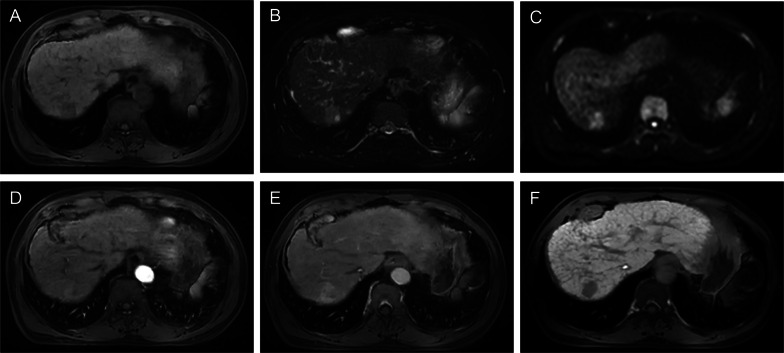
Images in a 62-year-old man with chronic hepatitis B virus infection. A–F Gadoxetate disodium–enhanced axial MRI scans show a 2.4-cm mass in segment VII (subphrenic location), which shows T1 hypointensity (A), mild to moderate T2 hyperintensity (B), marked hyperintensity on DWI (b = 800 s/mm^2^) (C), mild inhomogeneous enhancement on arterial phase image (D), obvious enhancement on portal venous phase (E), and demonstrates marked hepatobiliary phase hypointensity (F). The recurrence-free survival for this patient was 50 days
